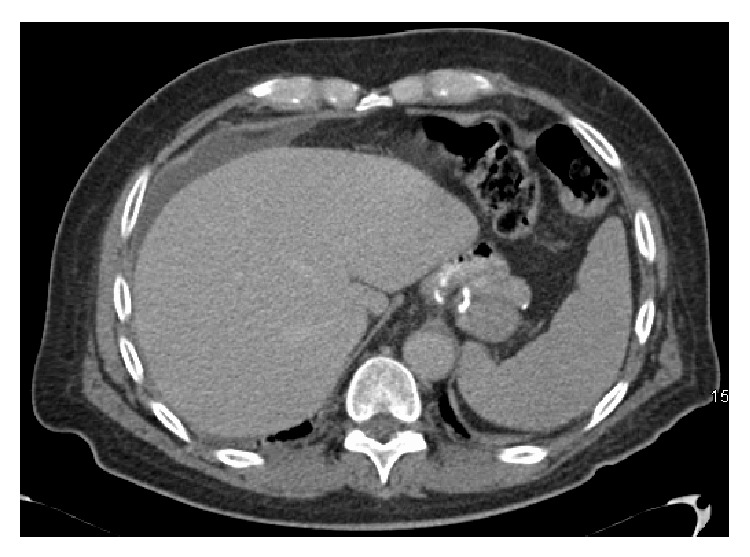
CT showing free fluid in the right paracolic gutter, no free air, and intact gastrojejunal anastomosis.
